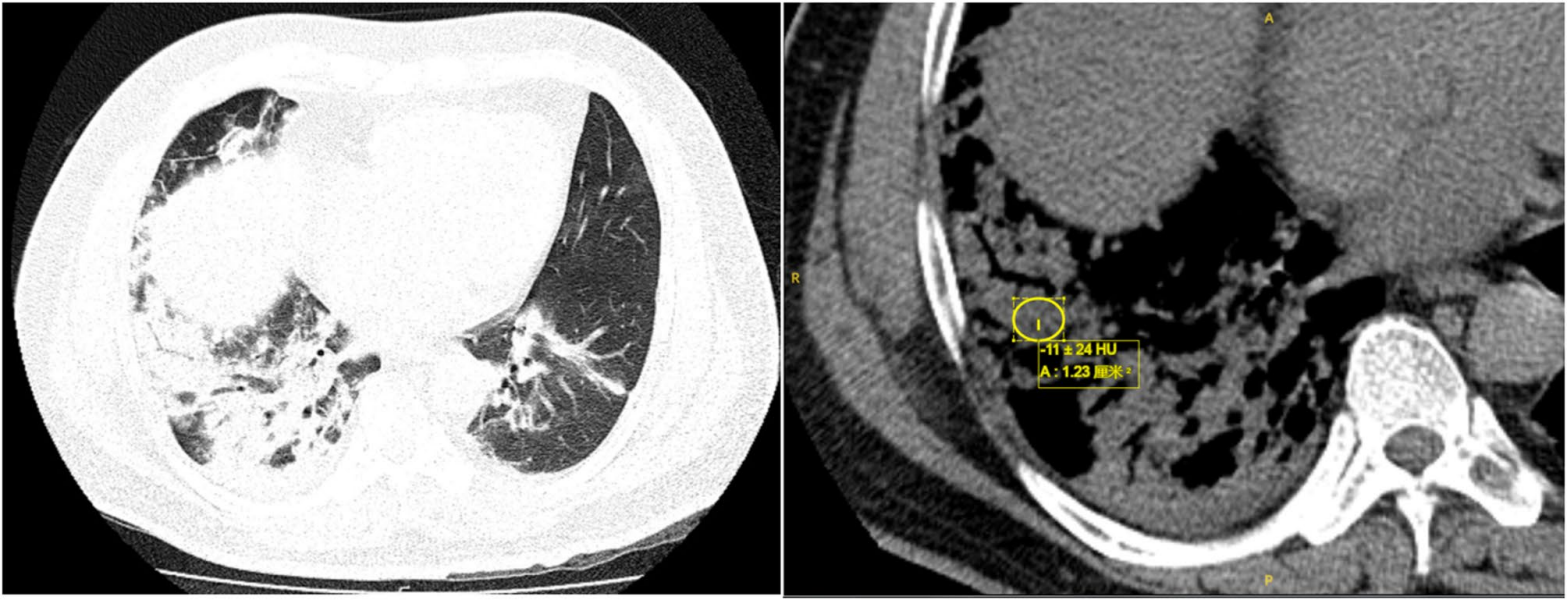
Chest CT showed multifocal consolidative opacities with areas of low attenuation on the first day of admission to Zhongshan Hospital
